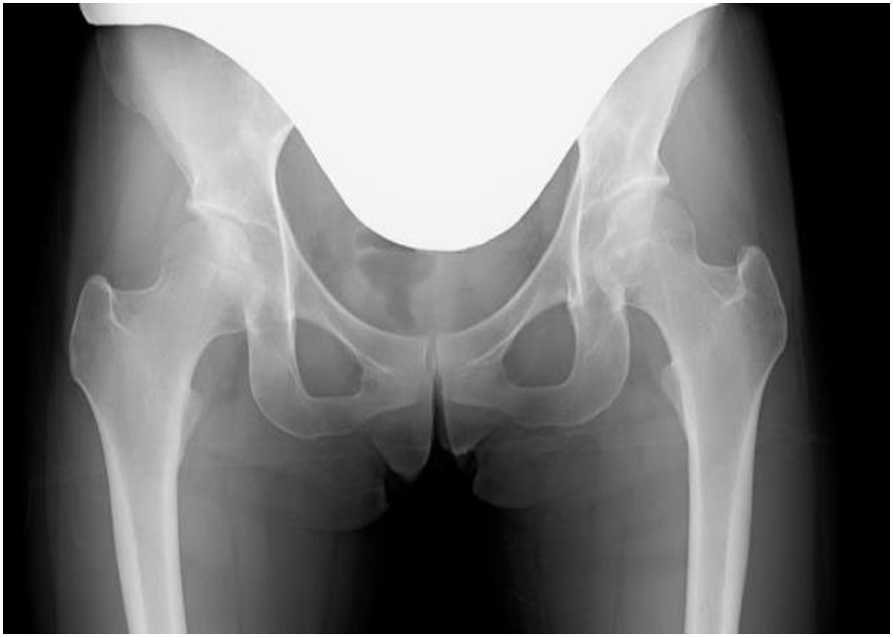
Plain radiograph of the bilateral hip joints after surgery. No narrowing of the joint space or collapse of the femoral head was noted.
